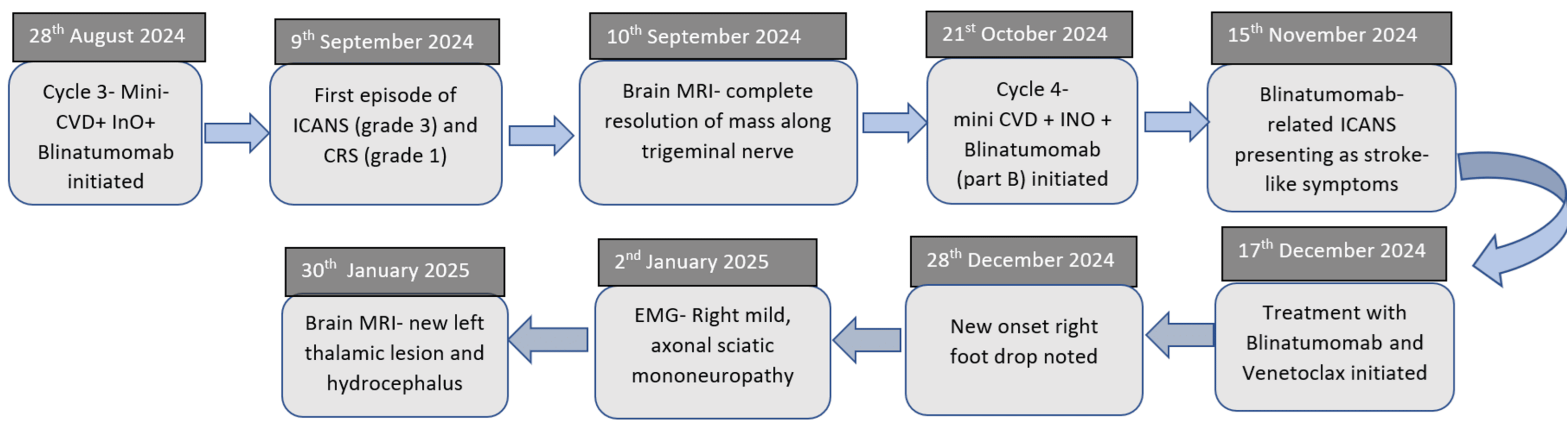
Chronological summary of key clinical events ICANS: immune effector cell-associated neurotoxicity syndrome; CRS: cytokine release syndrome; MRI: magnetic resonance imaging; EMG: electromyography
